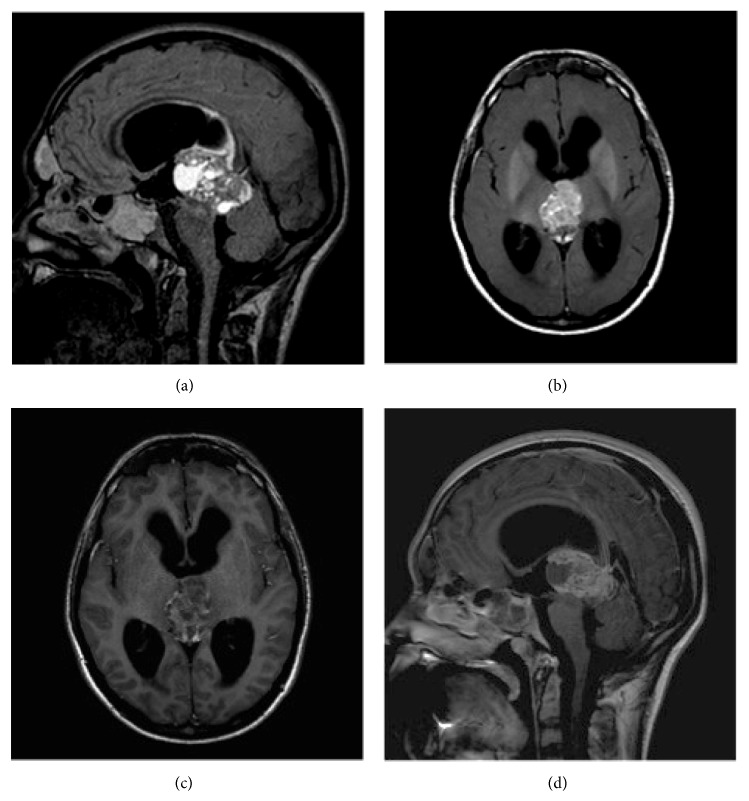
Sagittal FLAIR (a) shows solid and cystic portions, both containing high protein content. Axial T1 SE/MTC (b) shows more pronounced hyperintensity than the comparative T1 SE (c). T1 SE after gadolinium administration (d) confirms heterogeneous enhancement in the solid portion of the tumor and the cystic walls.
